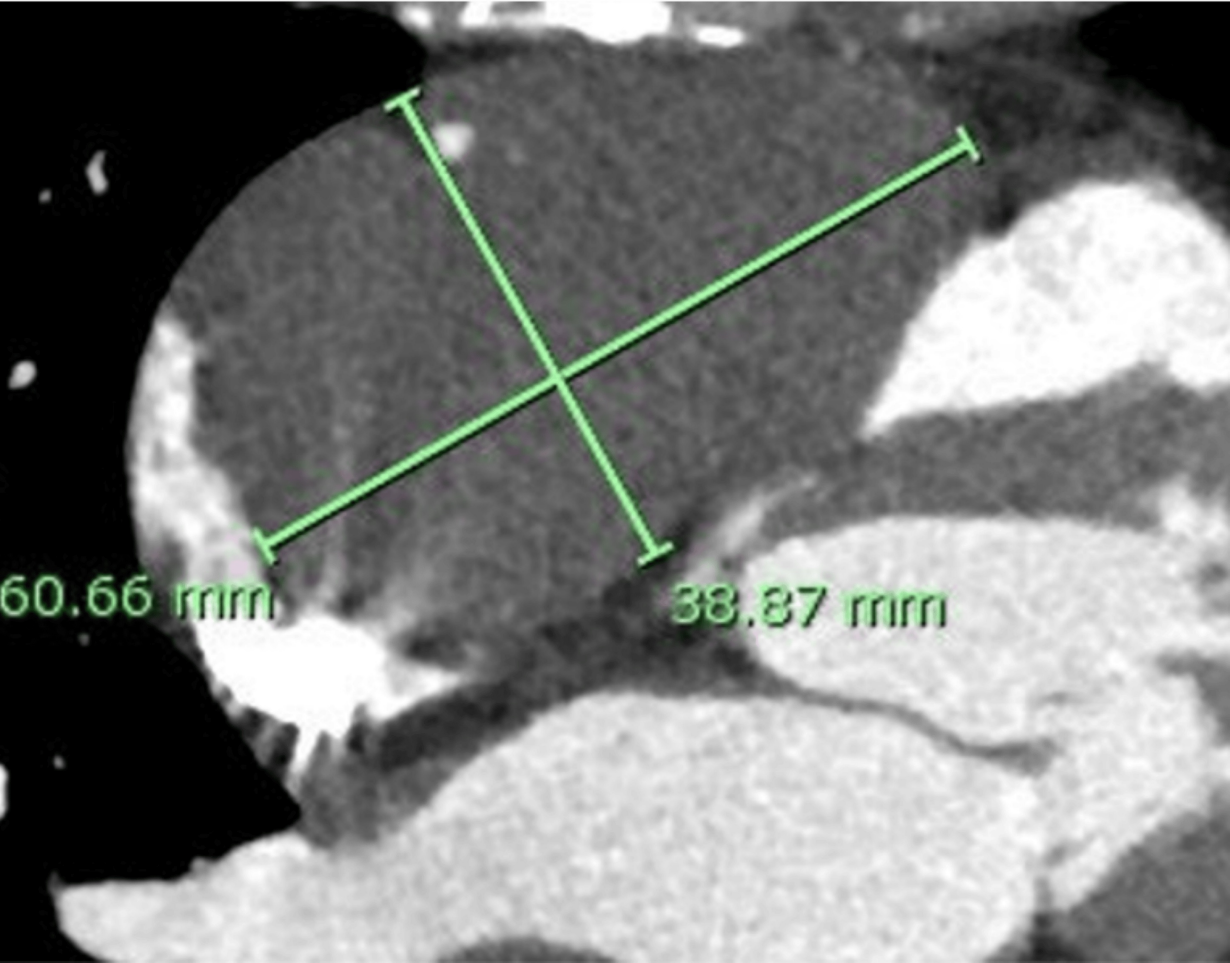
Preoperative computed tomography. Computed tomography imaging showed a low-attenuation mass on the anterior surface of the right atrium.
